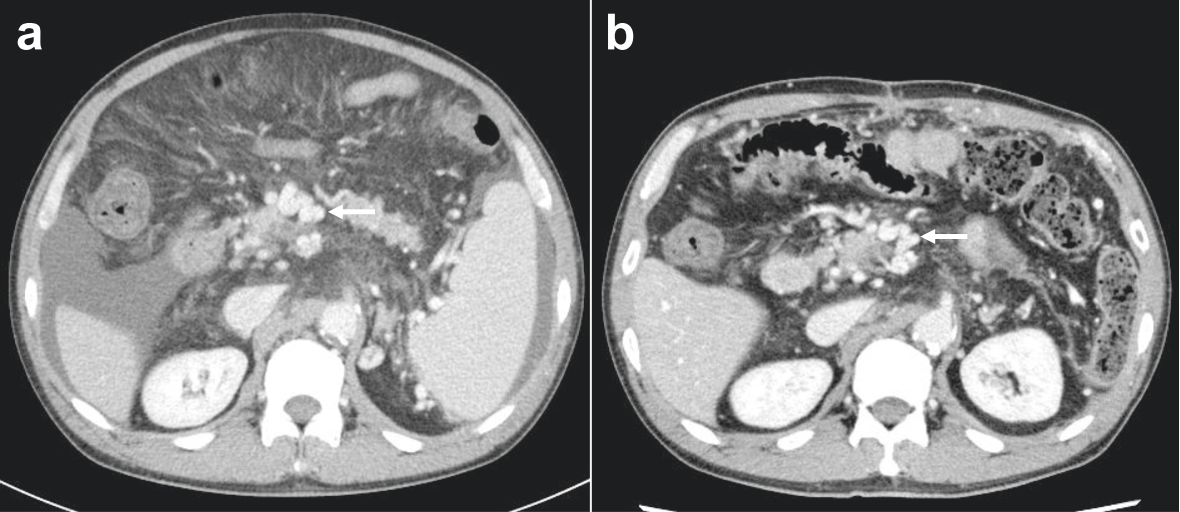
Preoperative CT (a) and postoperative CT (b). CT 3 months after the surgery showed disappearance of ascites and mesenteric edema. The dilatation of the mesenteric vein was reduced (*white arrow*)
